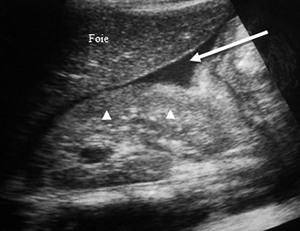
Ultrasound image of a hemoperitoneum in Morrison's lodge and kidney stage 1 injury. Hypoechoic collection (white arrow) in Morrison's lodge between the liver (*foie* in French) and the right kidney (white triangles) of echogenicity equal to the liver (stage 1 kidney disease).
